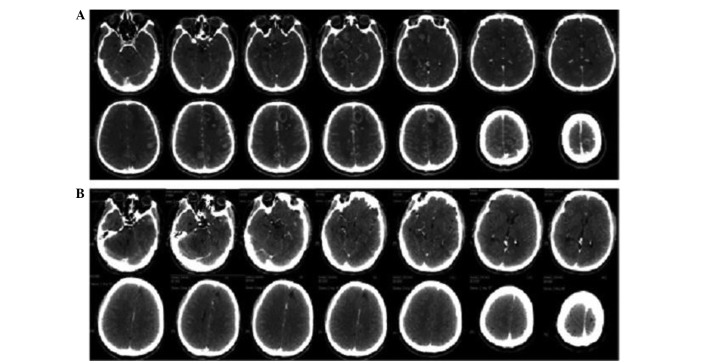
Multiple intracranial metastases (24 lesions). Computed tomography (CT) revealed that the tumors had disappeared following CyberKnife treatment. (A) CT prior to CyberKnife therapy (2010-12-04); (B) CT following CyberKnife therapy (2011-02-22).
